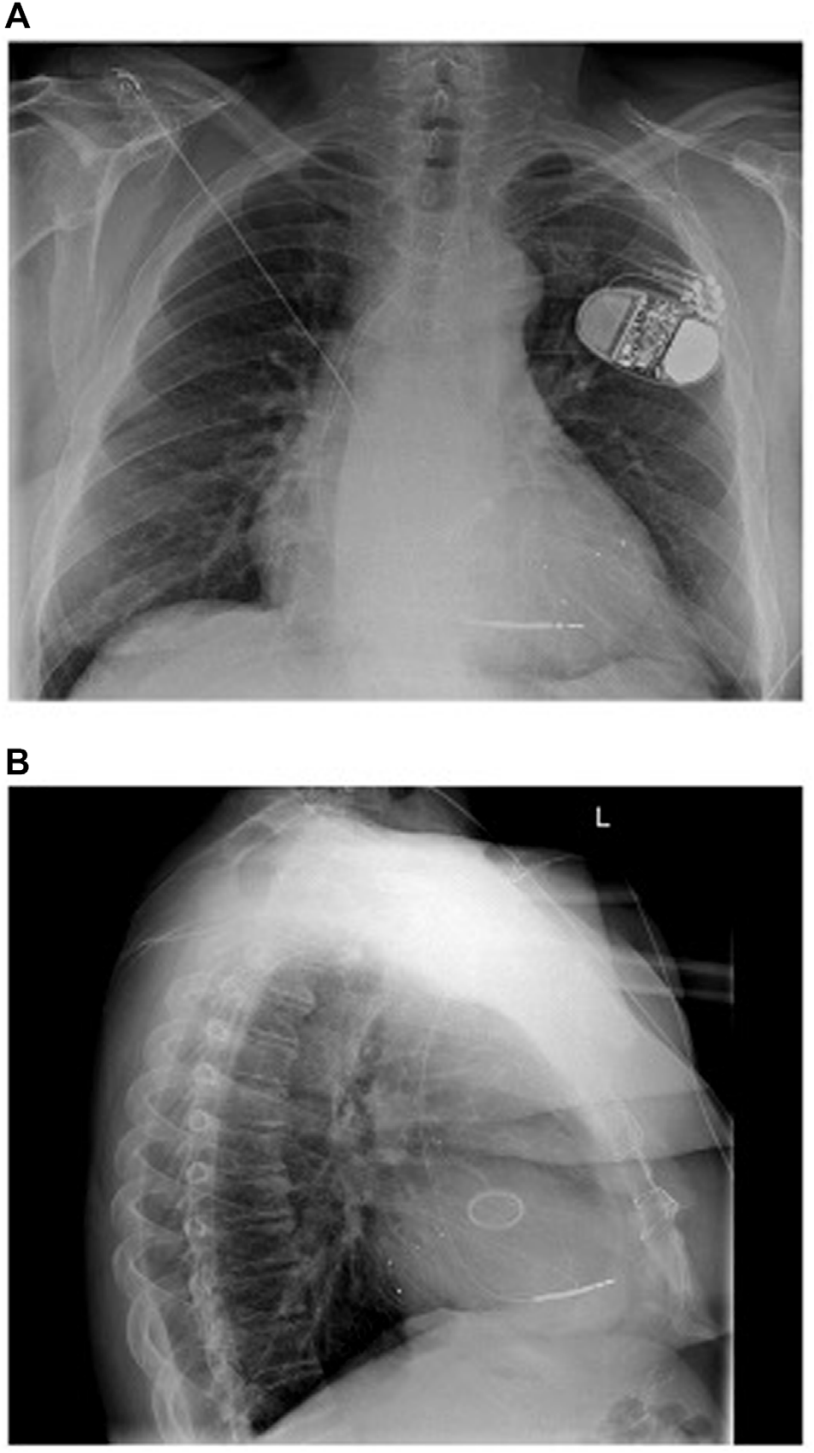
(A) Anteroposterior and (B) Lateral chest radiograph views 1 day post implant of triventricular CRT system with one LV lead in posterolateral vein and another in a lateral vein. Reproduced from reference 23, Gould et al., with permission.
